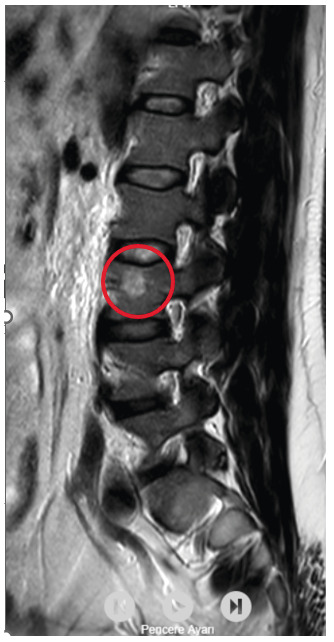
Sagittal T2-weighted images with fat suppression (T2 AG) show hyperintense signal intensity, indicating spondylodiscitis.
